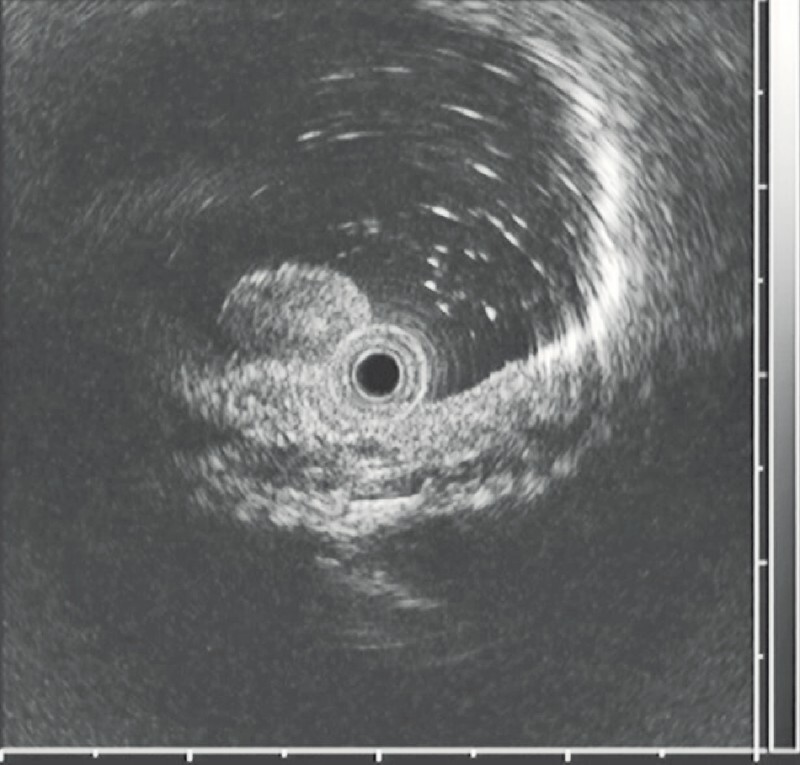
Endoscopic ultrasonography revealed the lesion was in the submucosa.
